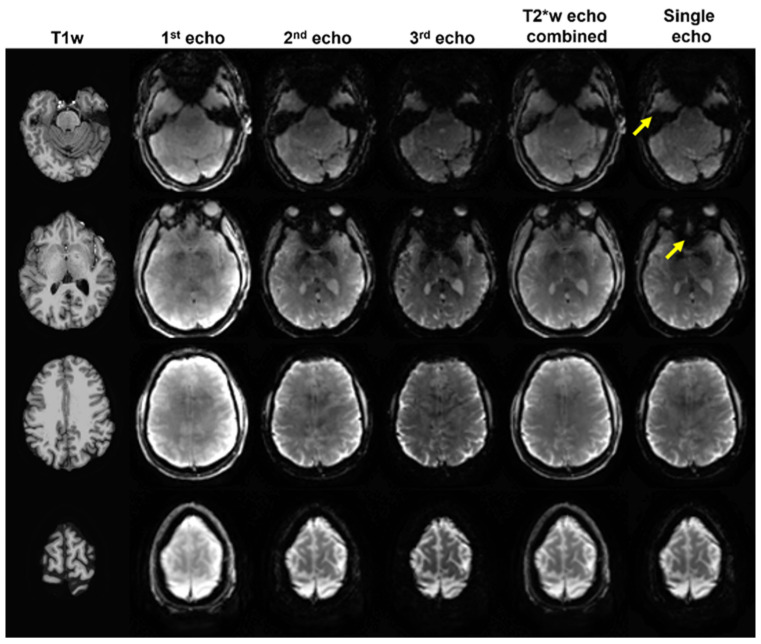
T1-weighted anatomical image and three echo time EPI images obtained on compact 3T. Echo combined EPI image shows signal intensity recovery in brain regions which suffers from signal dropout in single-echo EPI images as indicated. The yellow arrows indicate brain regions suffering from signal dropout with high magnetic susceptibility in EPI images.
